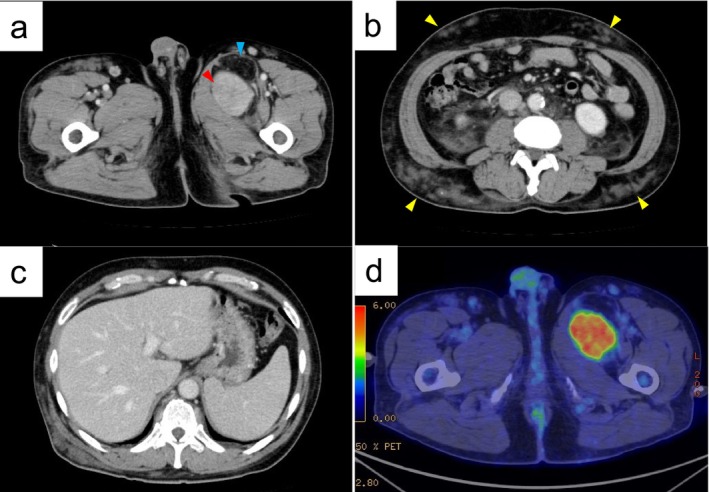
Contrast computed tomography (CT) findings. Borderline well‐defined mass lesion within the left thigh adductor muscle, with a 150‐mm large fatty dense area (blue arrow) and a lightly contrasted solid area (red arrow) (a). Multiple areas of soft density with indistinct boundaries within the subcutaneous fat of the trunk (yellow arrow) (b). Hepatosplenomegaly (c). Fluorodeoxyglucose positron emission tomography‐CT (PET‐CT) showing an accumulation with a maximum standard uptake value (SUVmax) of 6.84 in the solid area of the mass (d).
